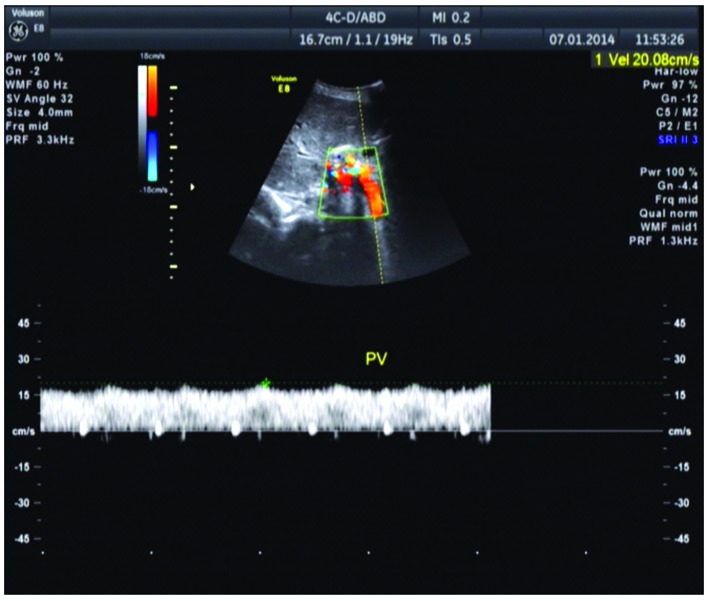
Color ultrasound of the upper abdomen showing diffuse disease of the hepatic parenchyma.
